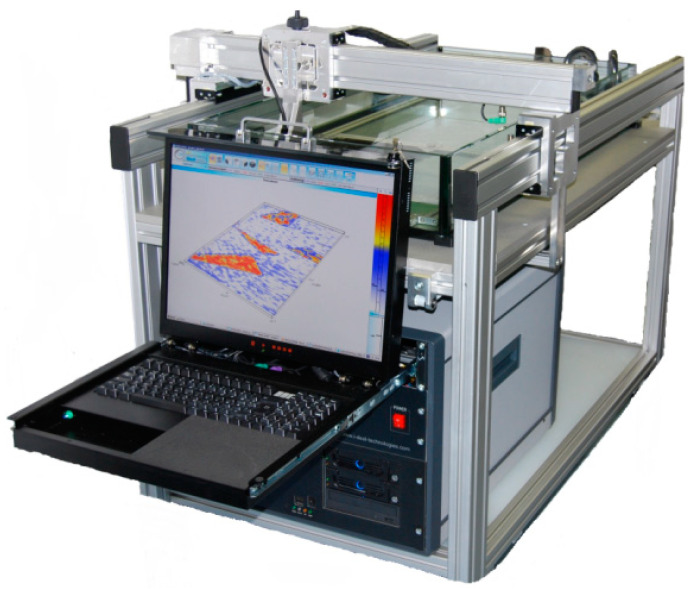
Multichannel ultrasonic inspection system: Idealsystem.
